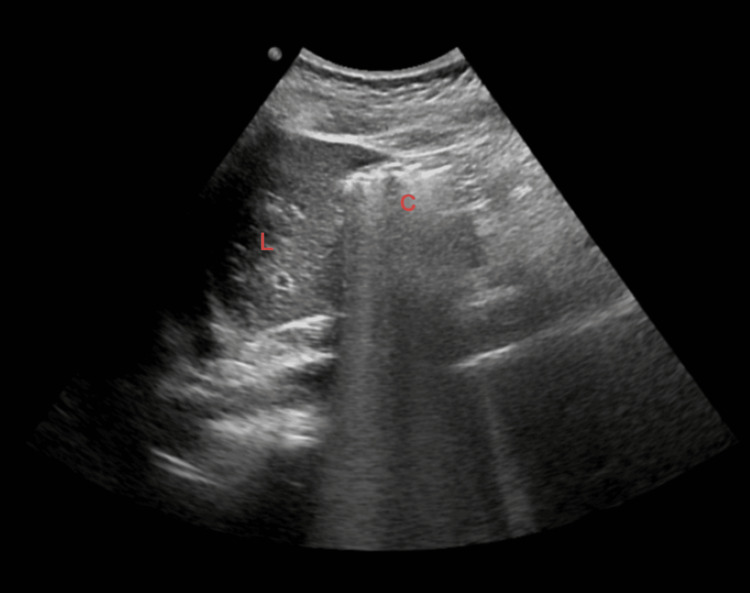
Initial ultrasound Abdominal ultrasound demonstrating non-visualization of the gallbladder in the gallbladder fossa. The echogenic focus, initially suspected to represent gallstones, was later determined not to correspond to a true gallbladder structure (L = liver, C = colon).
